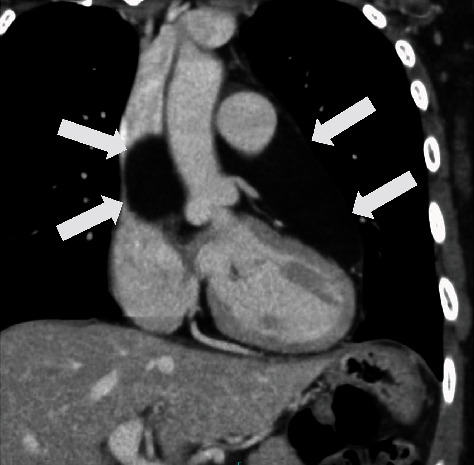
Preoperative enhanced computed tomography image. A mass on the posterior surface of the ascending aorta and main pulmonary artery is shown.
